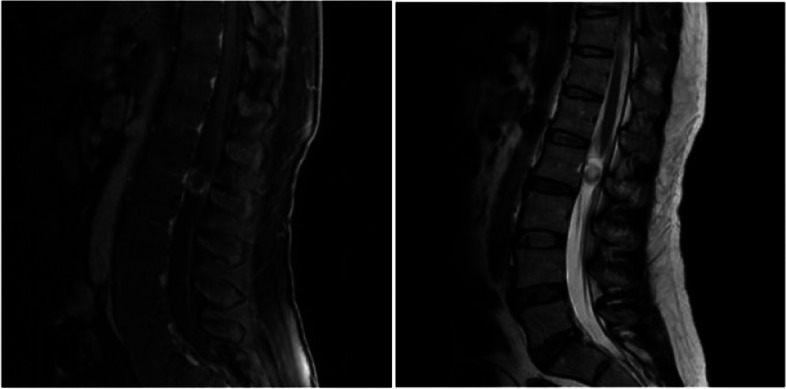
Preoperative lumbar MRI showed intraspinal space occupying lesions at L2 level
